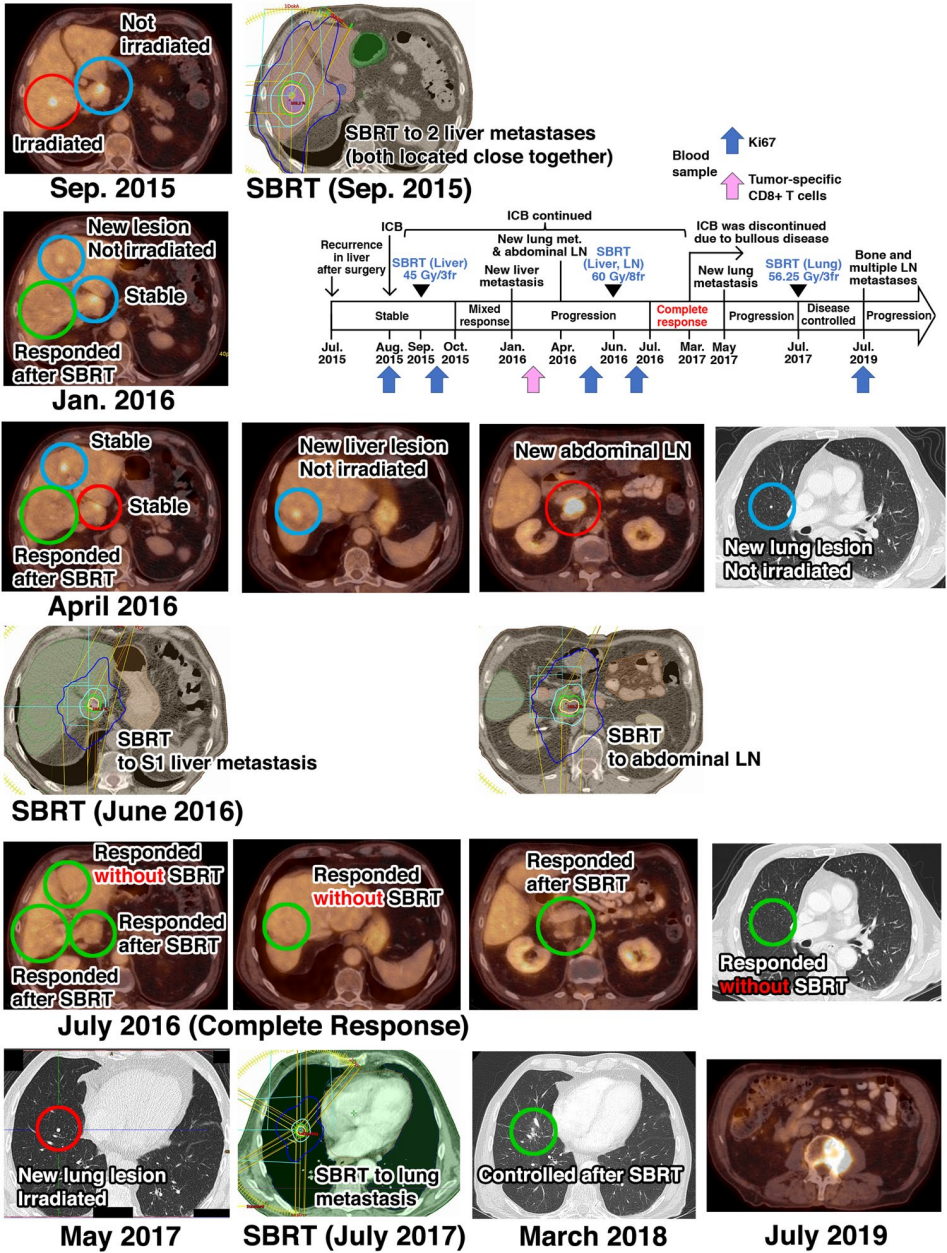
Disease and treatment course for patient 2 as well as FDG-PET/CTs and irradiation plans. The 1st SBRT in September 2015 was delivered in 3 fractions of 15 Gy every other day to the two largest lesions located close together in a non-central liver region. The 2nd SBRT in June 2016 was delivered in 8 fractions of 7.5 Gy over 2.5 weeks to the abdominal lymph node and a liver metastasis. The 3rd SBRT in July 2017 was delivered in 3 fractions of 18.75 Gy every other day to the new lung lesion. Irradiated lesions are highlighted by red circles, non-irradiated lesions by blue circles, and responding lesions by green circles. *ICB* Immune checkpoint blockade, *SBRT* stereotactic body radiation therapy, *LN* lymph node
